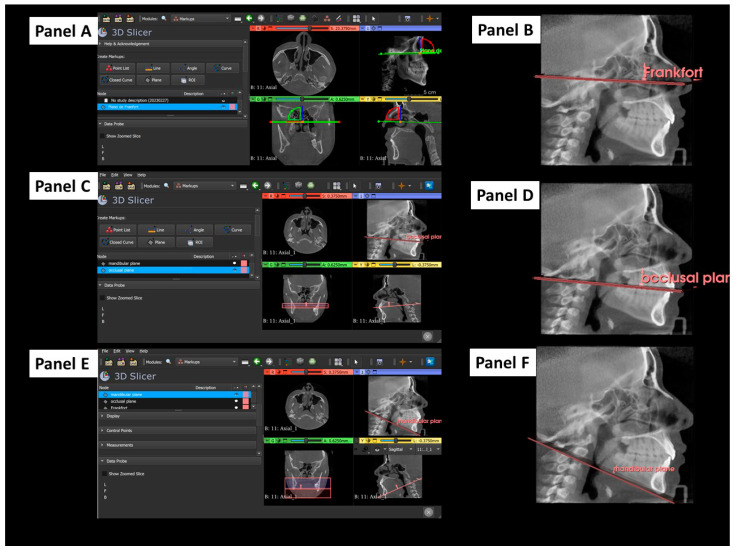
Cephalometric 3D landmarks in CBCT using 3D Slicer software. Panel (A): selection of the Frankfort plane. Panel (B): visualization of the Frankfort plane from the sagittal view. Panel (C): selection of the occlusal plane. Panel (D): visualization of the occlusal plane from the sagittal view. Panel (E): selection of the mandibular plane. Panel (F): visualization of the mandibular plane from the sagittal view.
